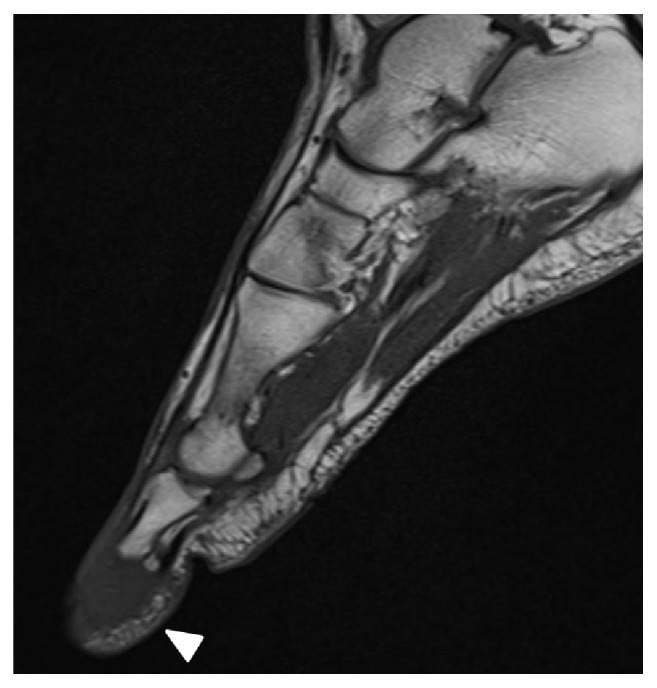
T1-weighted magnetic resonance imaging of the left foot shows low intensity in the first distal phalanx.
